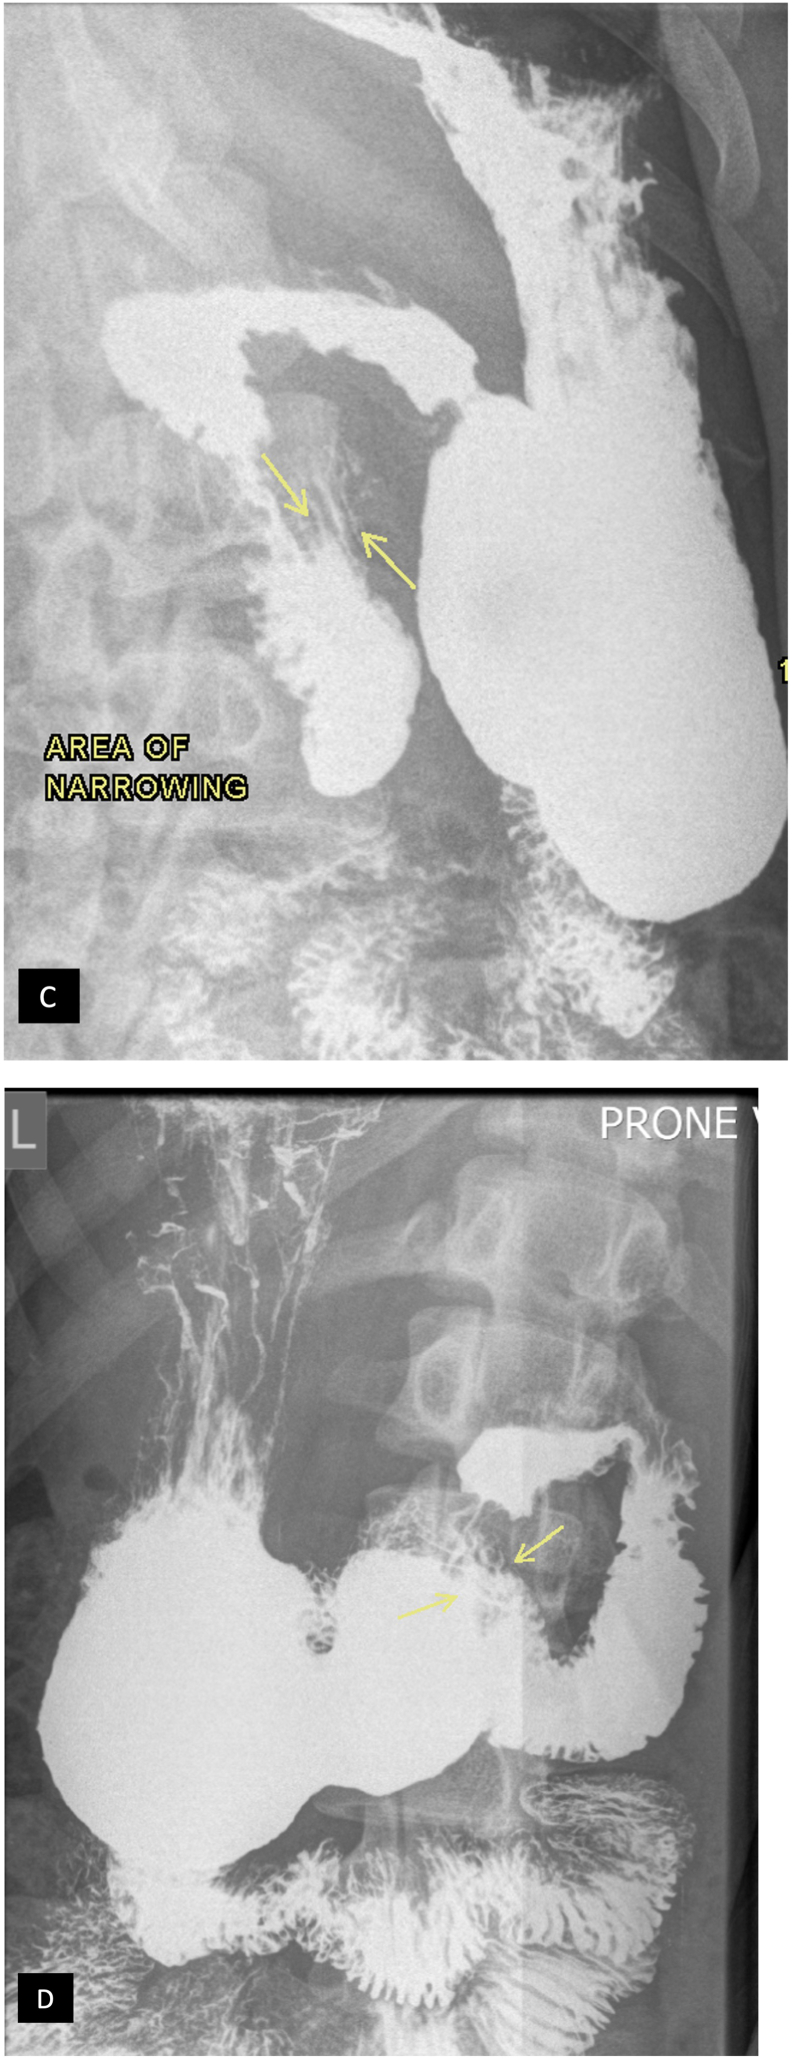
Barium meal follow on erect abdominal radiograph demonstrates in (C) dilated first and second portion of the duodenum with tapered narrowing at the third part of the duodenum (arrows). On repositioning the patient to prone (D), the oral contrast was seen passing into the third and fourth part of duodenum (arrows).
